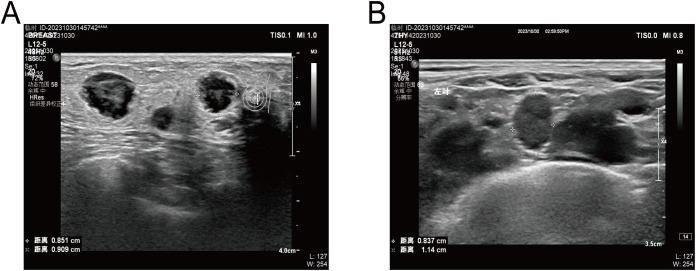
(A), Ultrasound showed several hypoechoic masses with blurred margins and irregular morphology. (B), Ultrasound showed enlarged lymph nodes in his left supraclavicular region.
